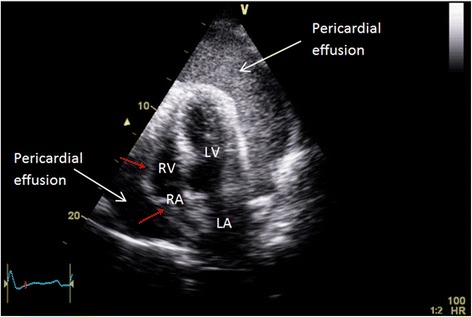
Apical four chamber view on initial presenation demonstating large pericardial effusion with tamponade causing compression of right heart chambers (red arrows). LV function was normal prior to pericardiocentesis
